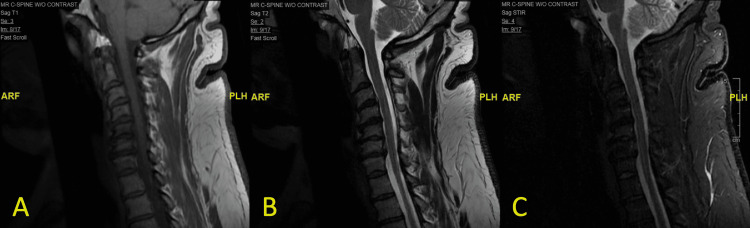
Repeat MRI of the cervical spine following empiric treatment Sagittal view of the cervical spine demonstrates moderate loss of disc space of the C5-C6 and C6-C7 intervertebral discs, unchanged from previous imaging. There is no vertebral osteomyelitis or discitis identified in the cervical spine on T1 (A), T2 (B), or short tau inversion recovery (STIR) (C) imaging.
